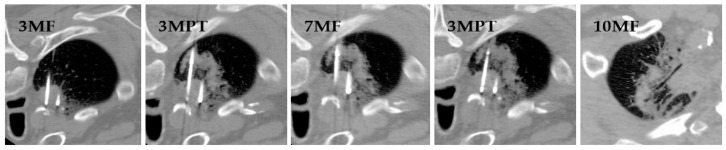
CT-visualization of a modified triple-freeze cryoablation protocol: 3 min freezing (3MF), 3 min passive thawing (3MPT), 7 min freezing (7MF), 3 min passive thawing (3MPT), and 10 min freezing (10MF). This is followed by active thawing and probe removal. Note that the patient was flipped from prone to supine position after 3 min freezing in the last image, as this is likely to decrease the risk of pneumothorax.
